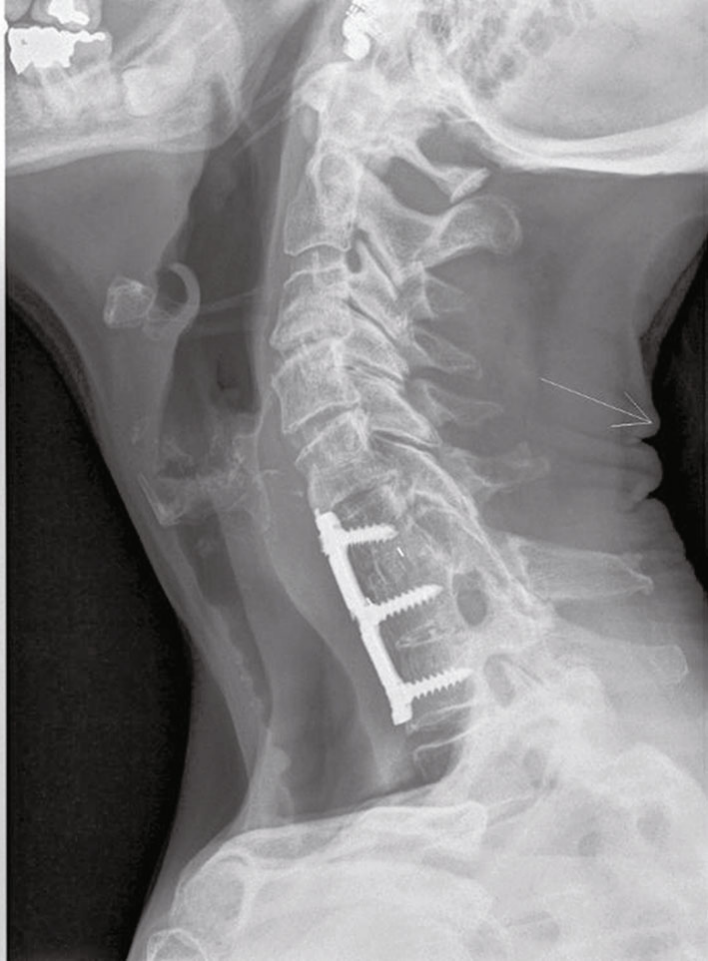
Postoperative X-rays (lateral view).
